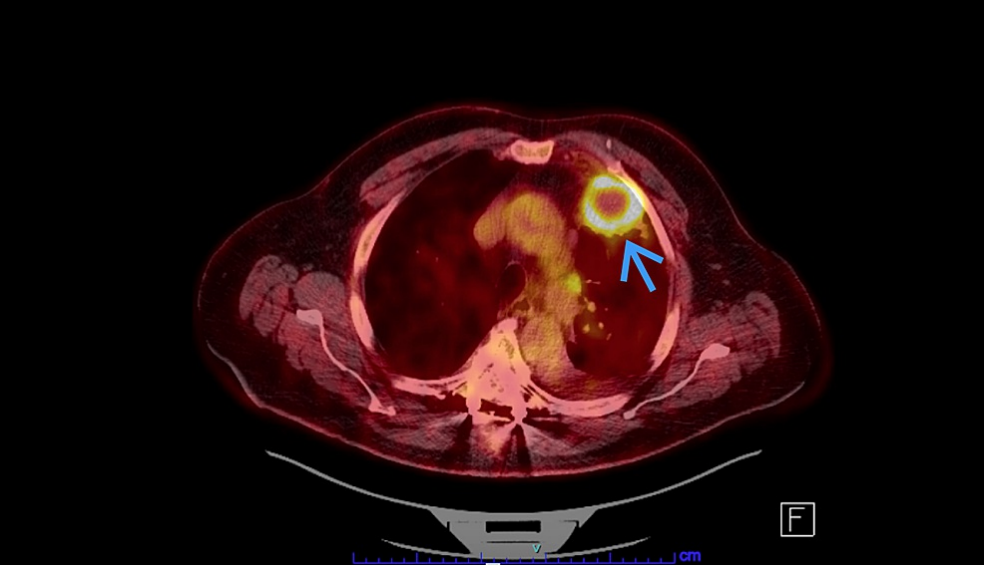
PET CT scan of the chest with intensely hypermetabolic necrotic mass in the anterior left upper lobe (Blue arrow)
